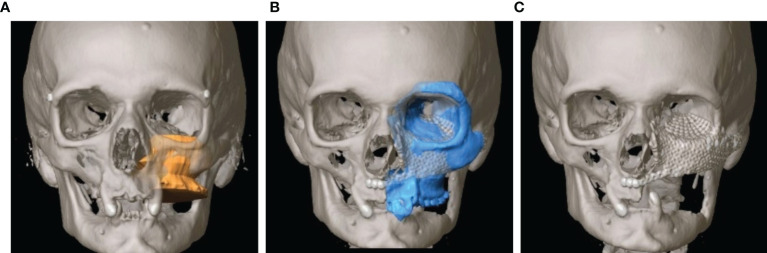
Virtual reconstruction planning using the principle of mirroring. (A) Segmented tumor of the left maxillary sinus with infiltration into the left floor of the orbit (yellow). (B) Template of the healthy right midface mirrored to the left (blue). (C) Postoperative CT shows the reconstruction of the orbit and the midface using two PSIs.
